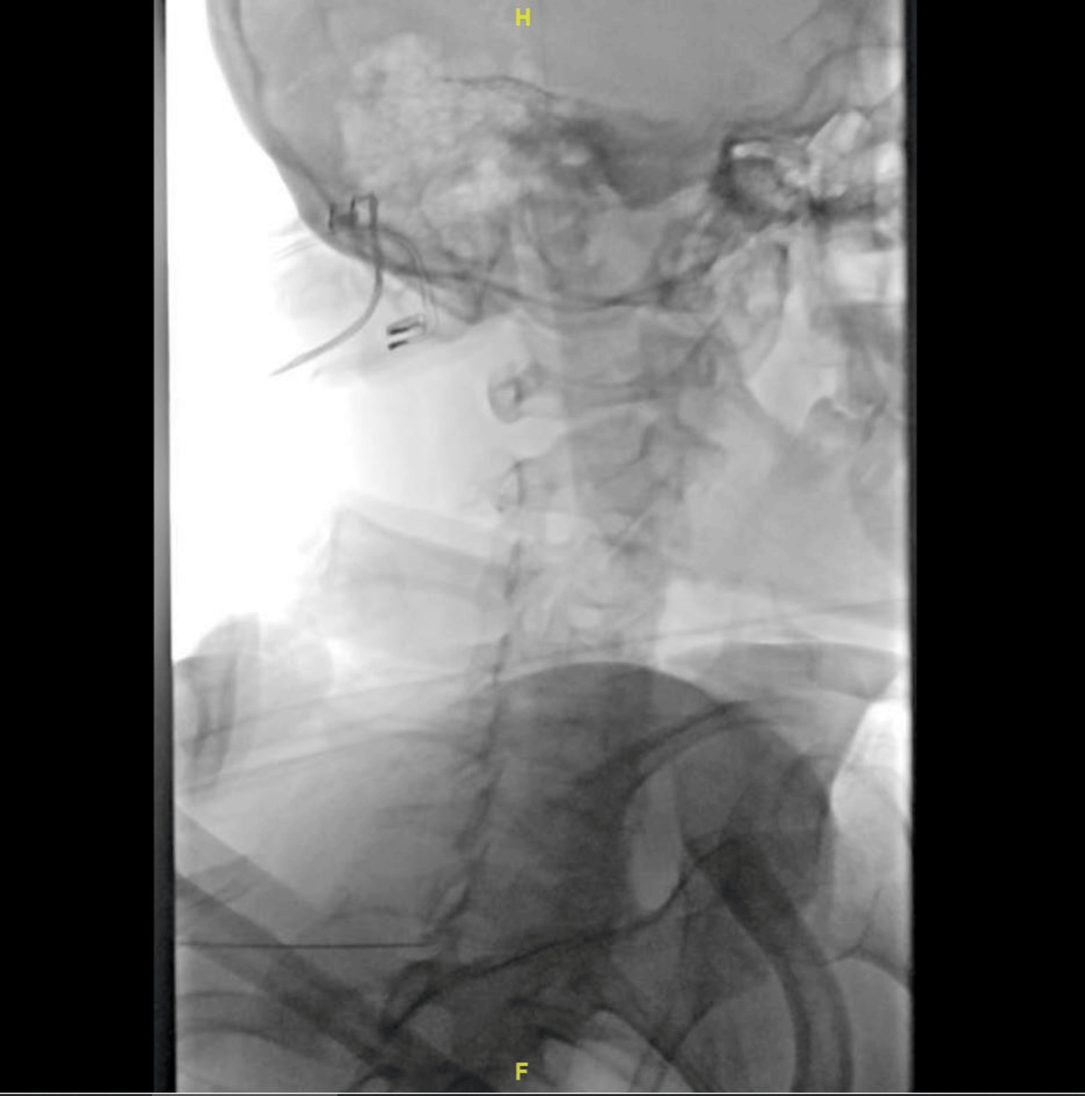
Cervical epidural blood patch performed at the C7/T1 level under fluoroscopic and CT guidance.
